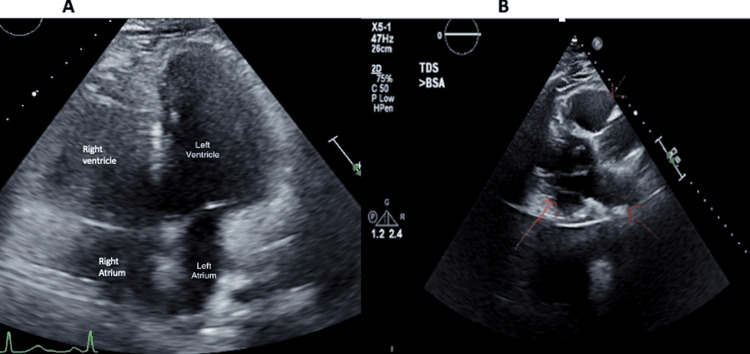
Transthoracic echocardiogram Mild concentric left ventricular hypertrophy with ejection fraction of 55-60%, mildly dilated left atrium, and right ventricle (labeled chambers in image A and red arrows in image B).
